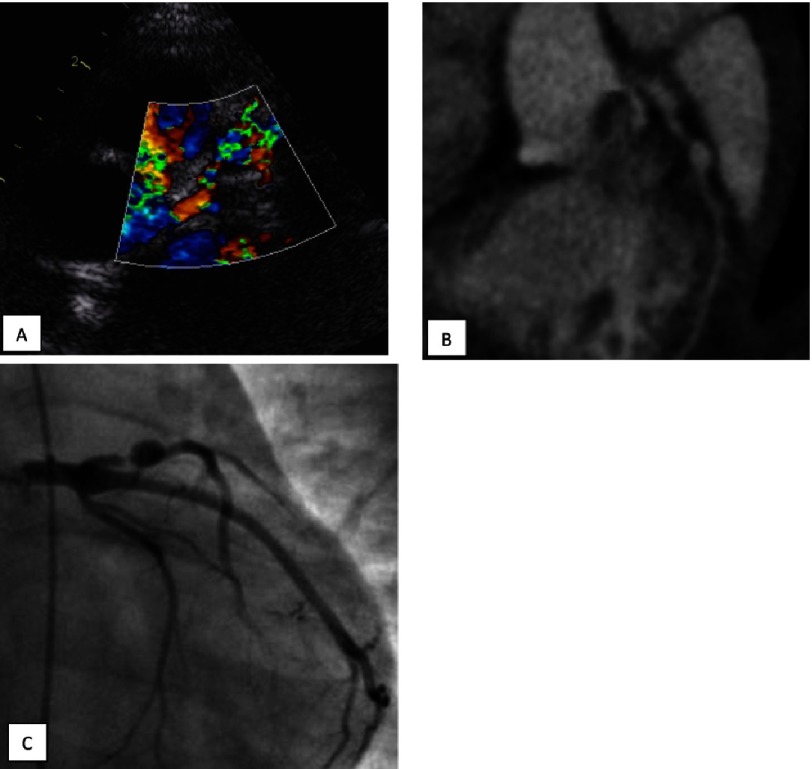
A subtotal stenosis at the entrance of an aneurysm of the LAD 2,5 years after the onset of KS in a boy who suffered KS at the age of 0,42 years. A – echocardiogram, color flow mode. B – MDCT, multiplanar reformation, C - invasive X-ray coronary angiography.
